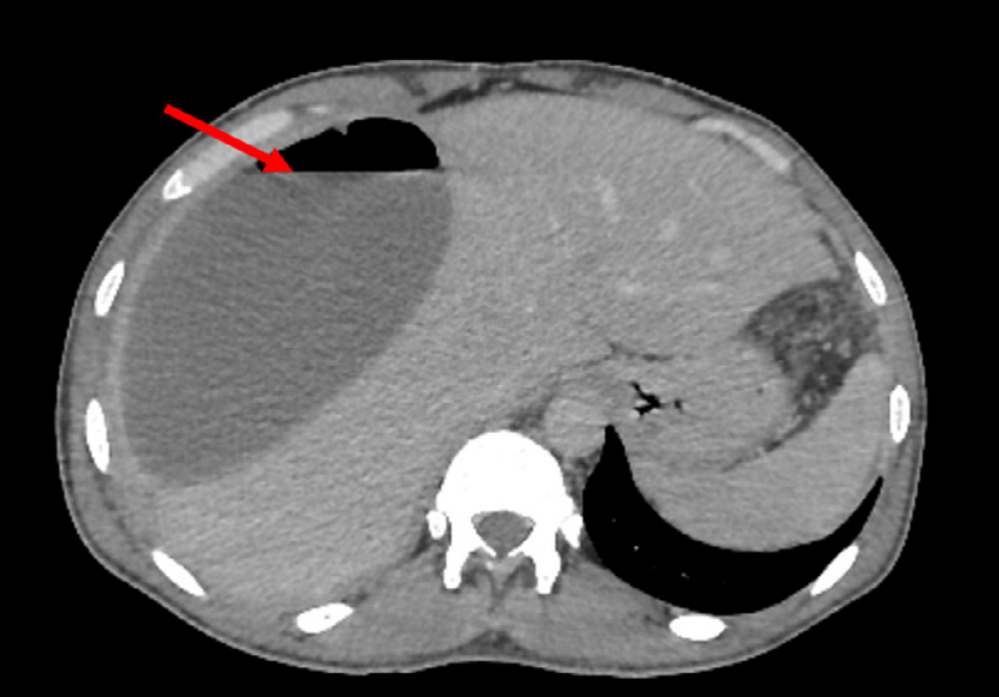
An axial slice of a CT image showing a hepatic abscess with a hydro-aeric level in the upper right quadrum of the abdomen (red arrow).
